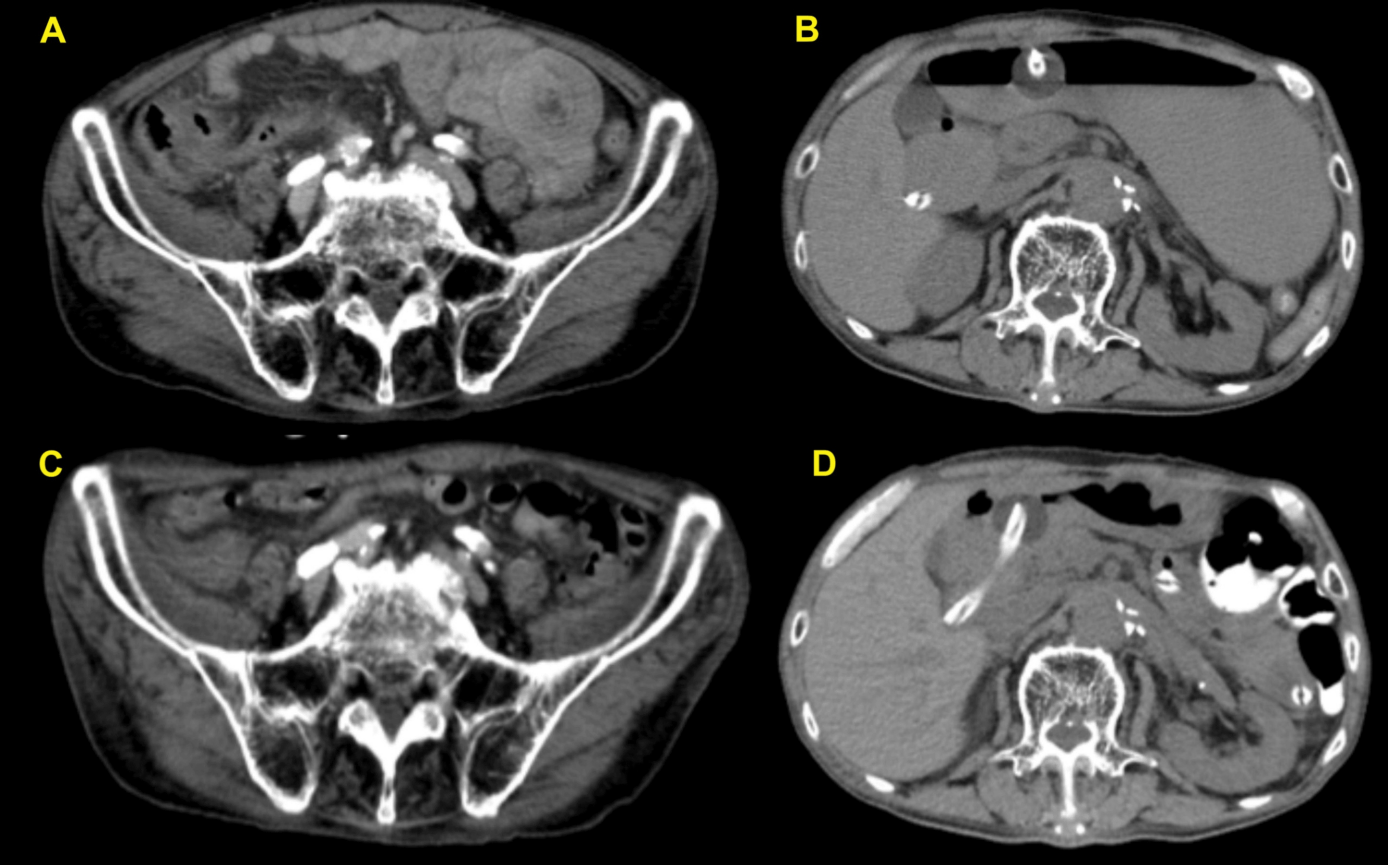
Abdominal CT scan over time (A, B) Before medication, CT showed jejunal intussusception and intestinal ileus. (C, D) On day 12 of medication, CT showed that jejunal intussusception and ileus improved. CT: computed tomography
